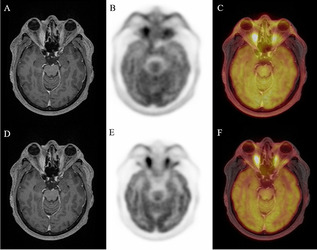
Transaxial MRI (panels A, D), PET (panels B, E), and fused slices of a 34-year-old patient with severe GO reveal pronounced orbital tissue hypertrophy, corresponding with high and extensive bilateral ^18^F-FDG uptake, both on early (panels A–C) and late static images (panels D–F)
